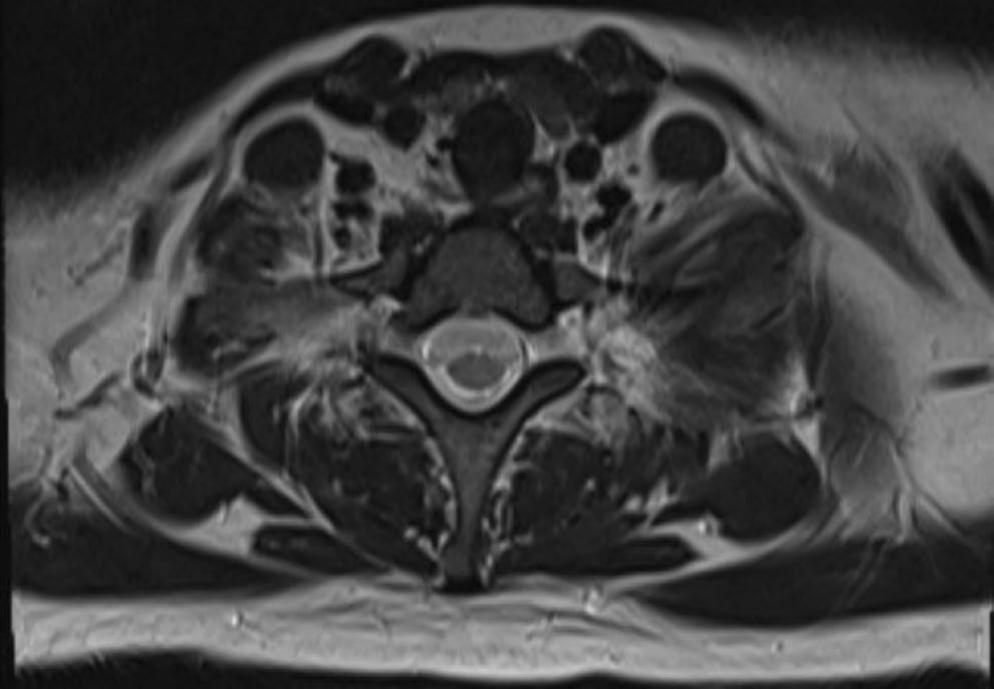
Cervical spine MRI T2‐weighted axial view at C7‐T1 level (normal).
